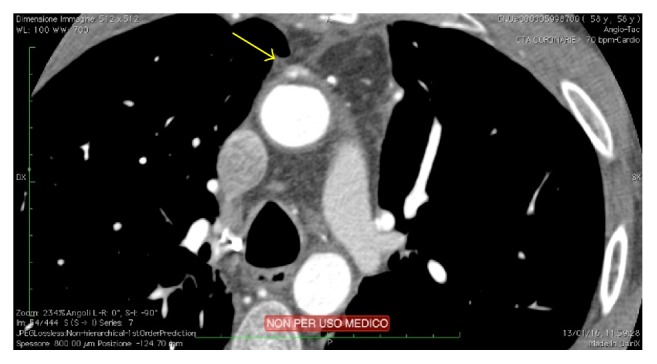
Thoracic CT scan of the patient two months after OPCAB. The yellow arrow points at a localized anterior wall disruption on ascending aorta, at the site of aortic punching.
